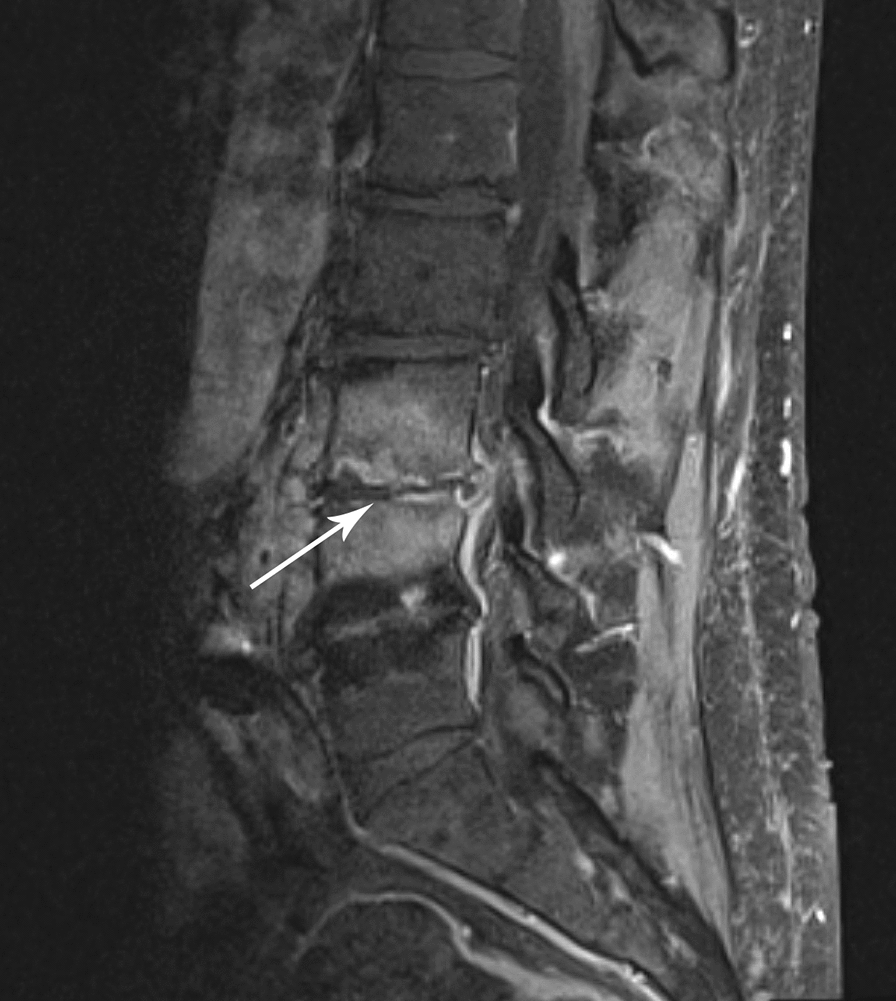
Magnetic resonance imaging (MRI) of the lumbar spine demonstrating discitis and vertebral osteomyelitis. T1 post-contrast sagittal MRI demonstrating enhancement at the L3–4 intervertebral disc space with erosions in the adjacent endplates (arrow)
